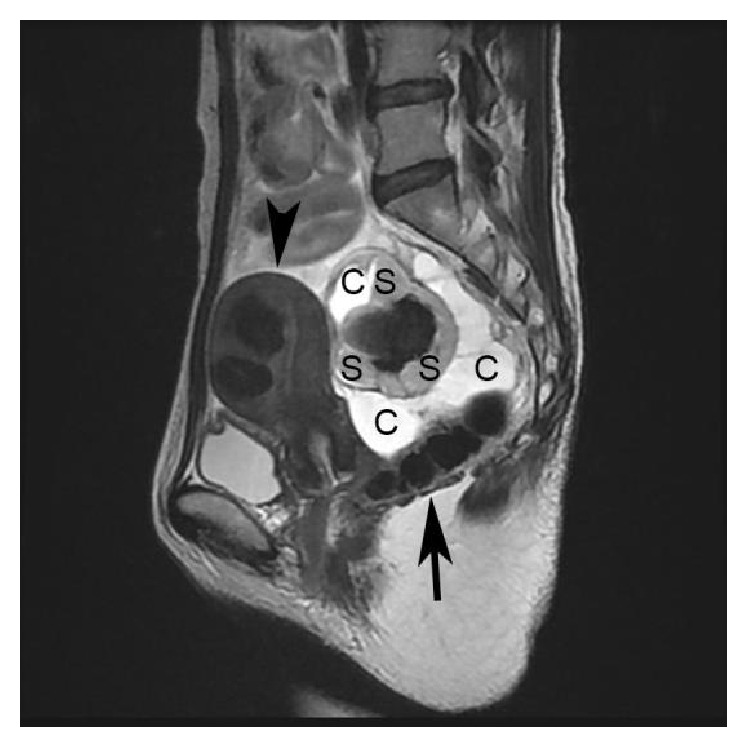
The rectovaginal mass on pelvic MRI (T2-weighted, sagittal line). The mass with multilocular cyst (indicated by C) and solid (indicated by S) components and a maximal diameter of 9 cm locates in the rectovaginal pouch. The uterus is indicated with an arrowhead, and the rectum is indicated with an arrow.
